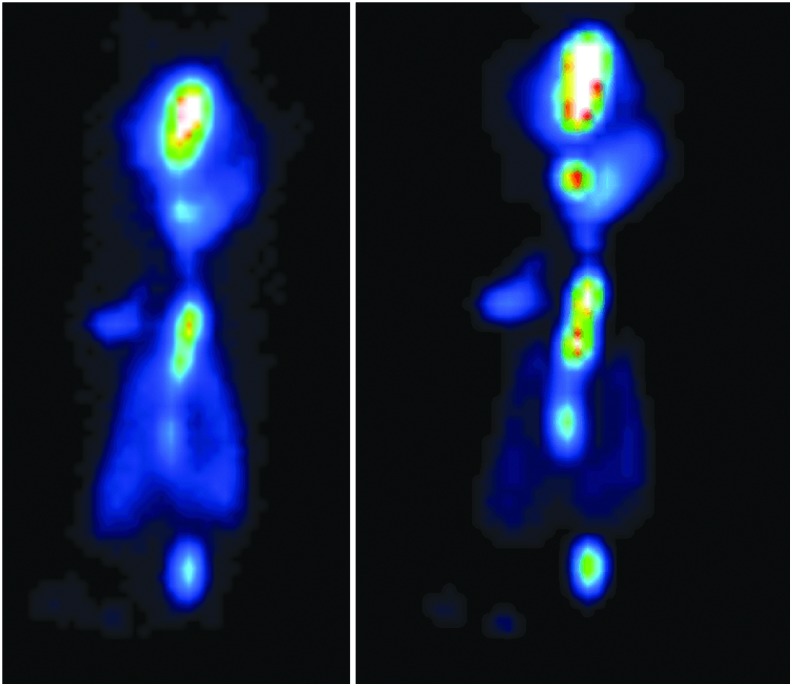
Planar (left) and SPECT (right) images from NHP-1 for the nCPAP circuit delivery.
